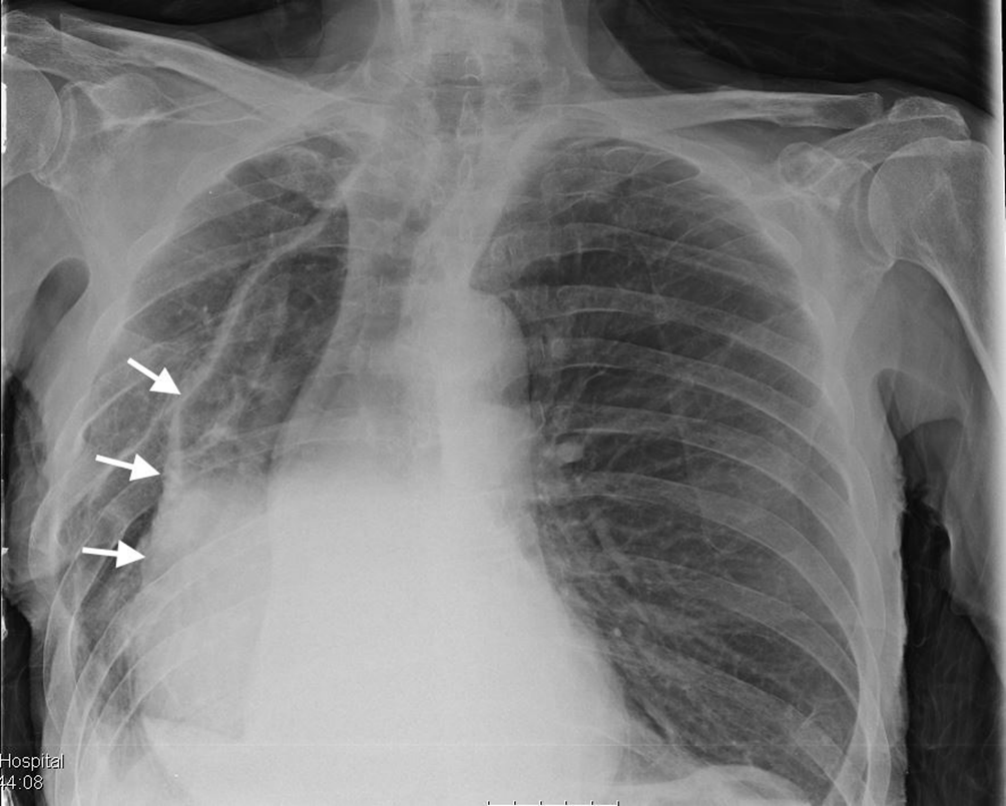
AP erect plain film chest radiograph showing the tubal stomach in the mediastinum following gastric pull-up procedure (arrows). AP, anteroposterior.
